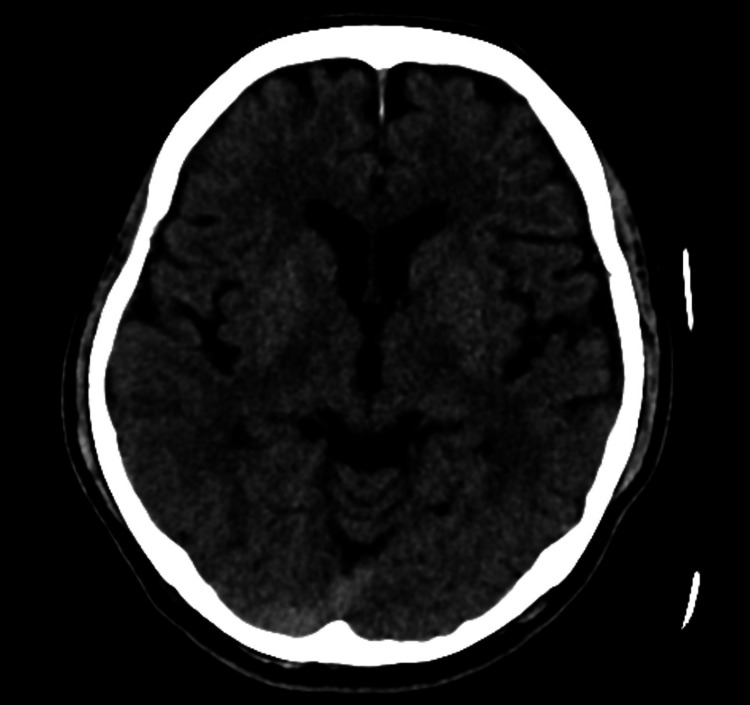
Plain CT brain revealed no acute intracranial hemorrhage, territorial infarct, or mass effect CT: computed tomography
